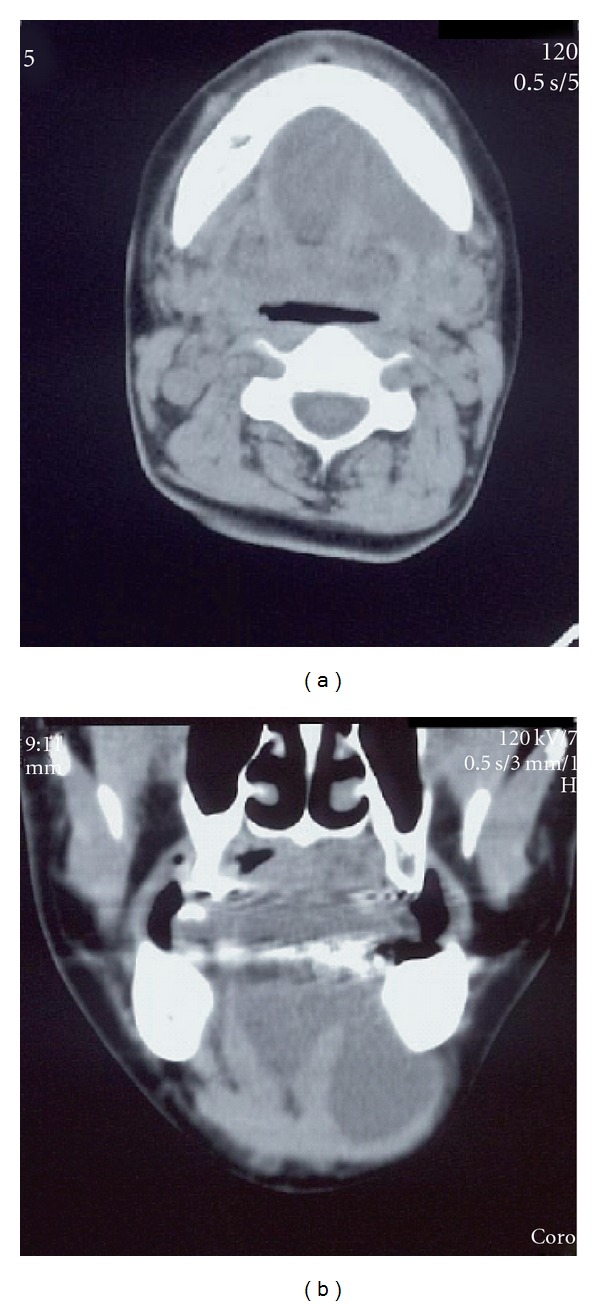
CT images. Axial (a) and coronal (b) images. CT revealing a mass of low density extending from the floor of the mouth to the left submandibular region.
